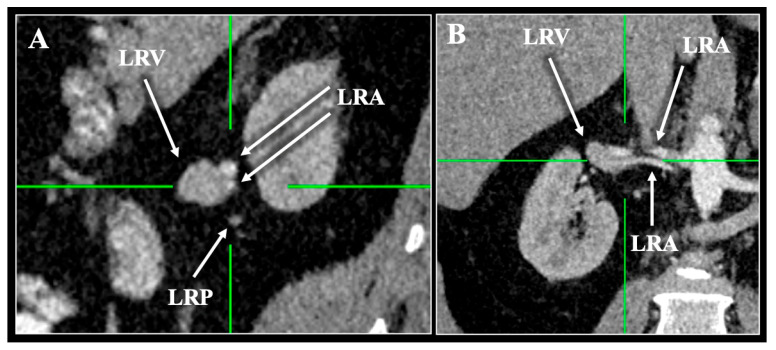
Contrast-enhanced computed tomography (CT) scan of a 48-year-old male patient demonstrating the Vein (V)—Artery (A)—Artery (A)—Pelvis (P) variation. Multiplanar reconstruction with sagittal (A) and coronal (B) slices. Two left renal arteries (LRAs) and a single renal vein (LRV) enter and exit the hilum. LRP—left renal pelvis. R—right, L—left, S—superior, I—inferior.
